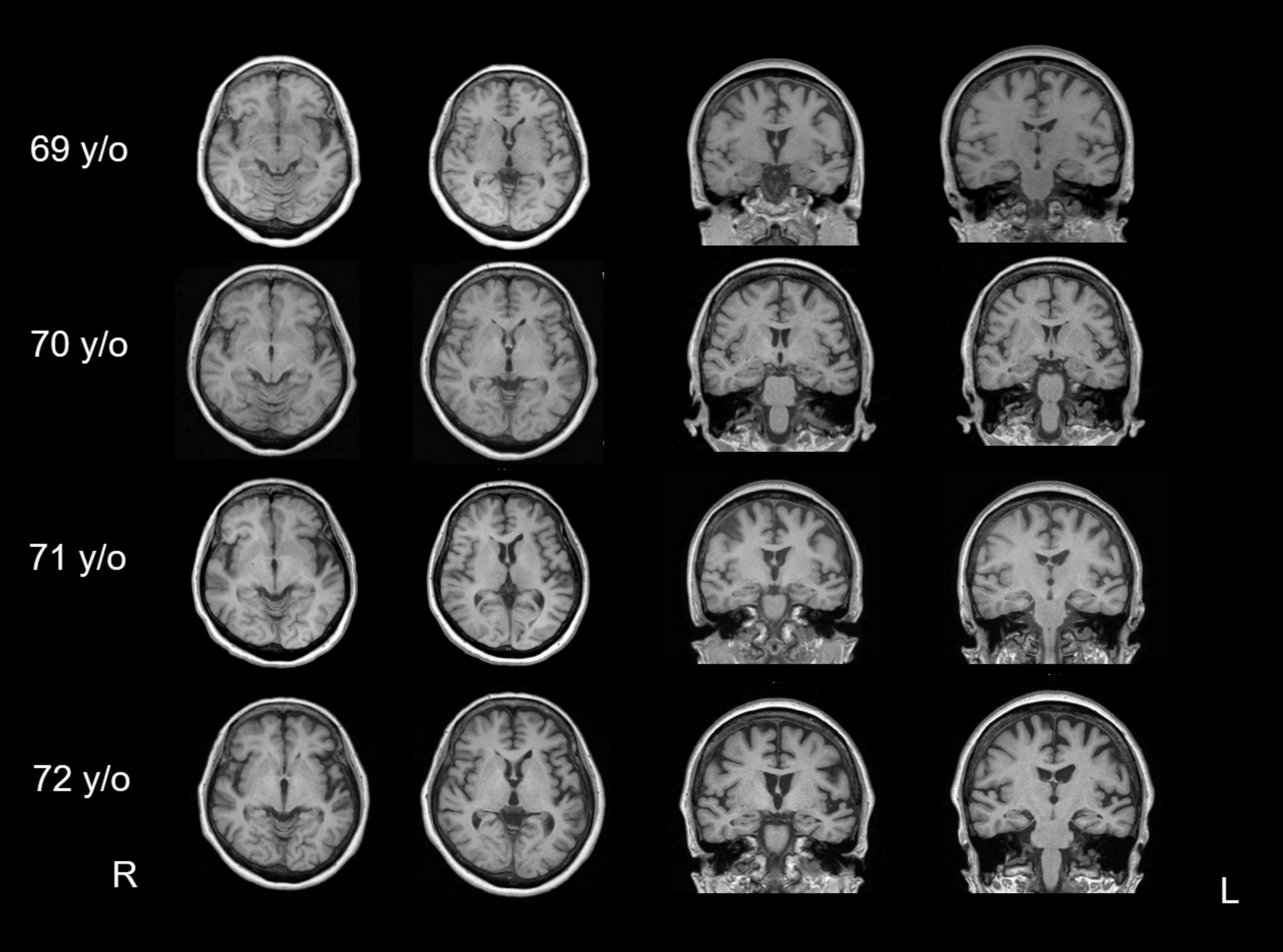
Serial T1 brain magnetic resonance images. L, left; R, right; y/o, years old
